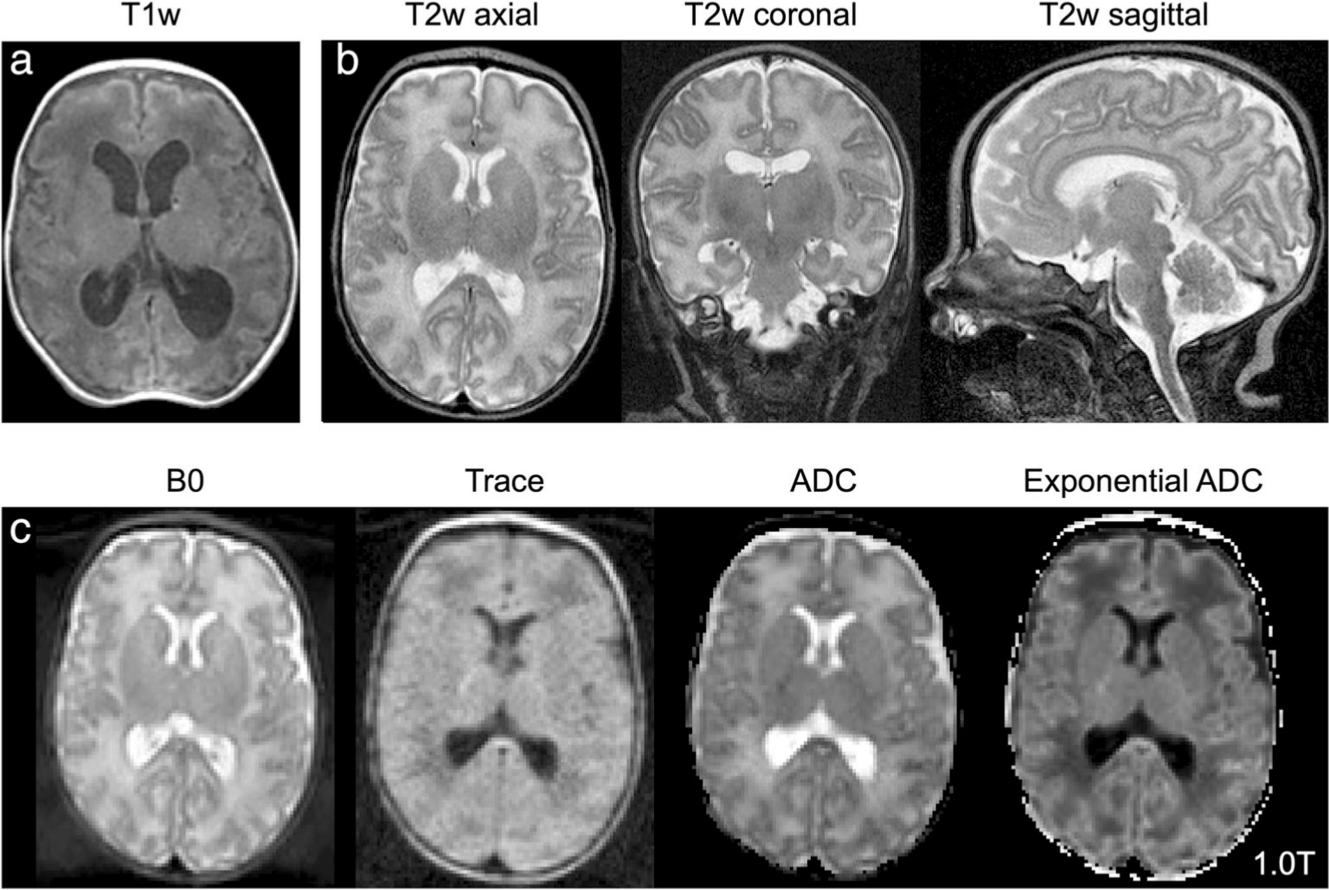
Images from pediatric patient imaged at 1T. (a) T1-weighted, (b) T2-weighted (left to right: axial, sagittal, coronal acquisitions), and (c) diffusion imaging (left to right: B0, trace, apparent diffusion coefficient [ADC], exponential ADC). Images provided courtesy of Wendy Slatery and John Posh, Aspect Imaging.
